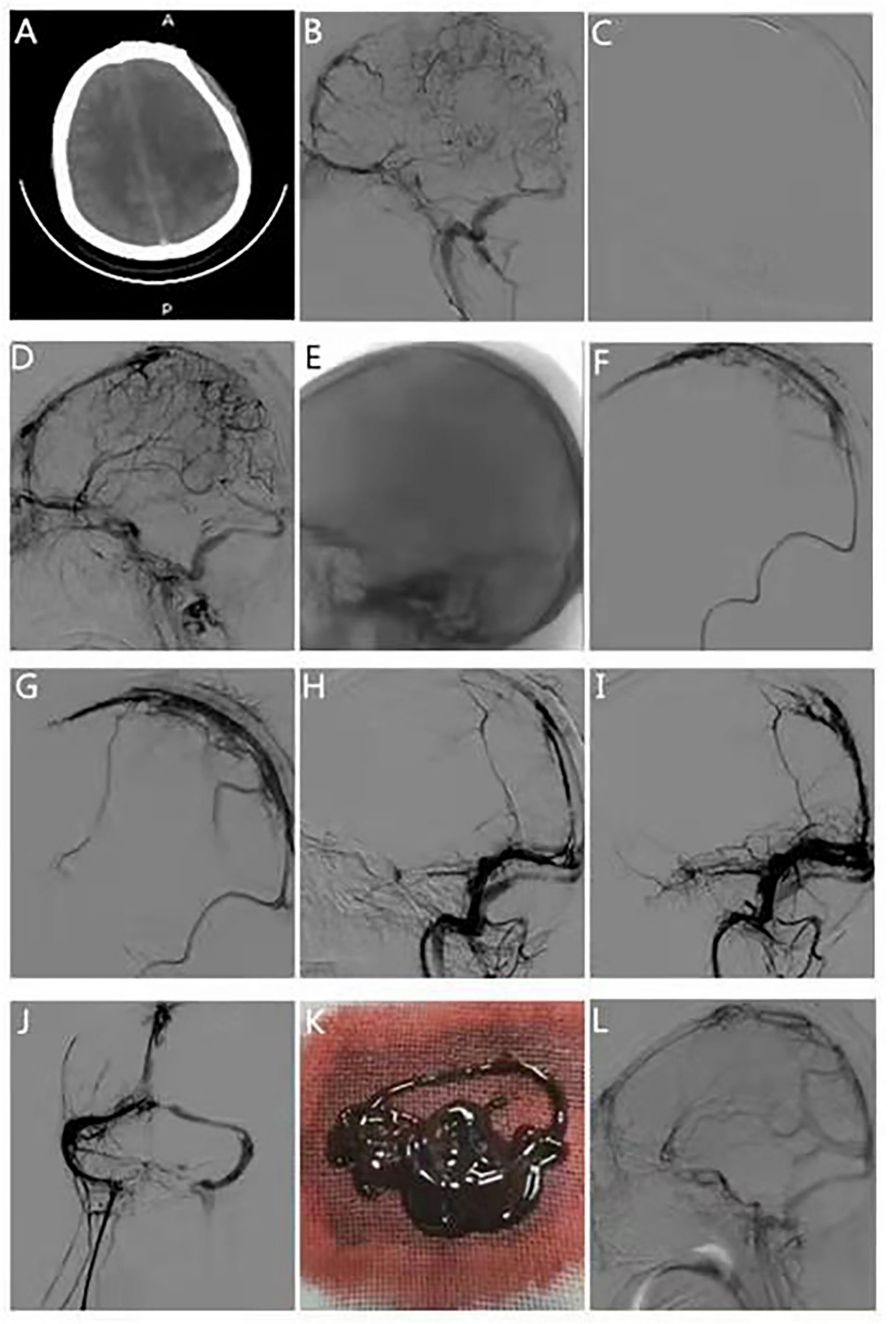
Typical case 2. The patient was a 29-year-old woman transferred from the outside hospital due to sudden headache, hemiparesis, coma, and limb twitching for 1 day. (A) Computed tomography scan of the head on admission suggests left parietal hemorrhage. (B) DSA suggests occlusion of the superior sagittal sinus, left transverse sinus, and sigmoid sinus. (C) Stent retrieval was performed from the distal part of the superior sagittal sinus. (D) The distal aspect of the superior sagittal sinus appears better than the anterior. (E) The intermediate catheter is delivered to the superior sagittal sinus midshaft using stent anchoring. (F) Thrombus retrieval in the middle segment of the superior sagittal sinus was performed using the solumbra technique. (G) The middle section of the superior sagittal sinus is better developed than before, but there is still proximal stenosis. (H) Stent retriever was performed to the proximal end of the superior sagittal sinus. (I) The posterior of the superior sagittal sinus appears better than the anterior. (J) Intravenous retrograde contrast suggests improved visualization of the superior sagittal, bilateral transverse, and sigmoid sinuses. (K) Intraoperative retrieved thrombus. (L) Review of DSA 90 days postoperatively suggests good visualization of the superior sagittal sinus.
